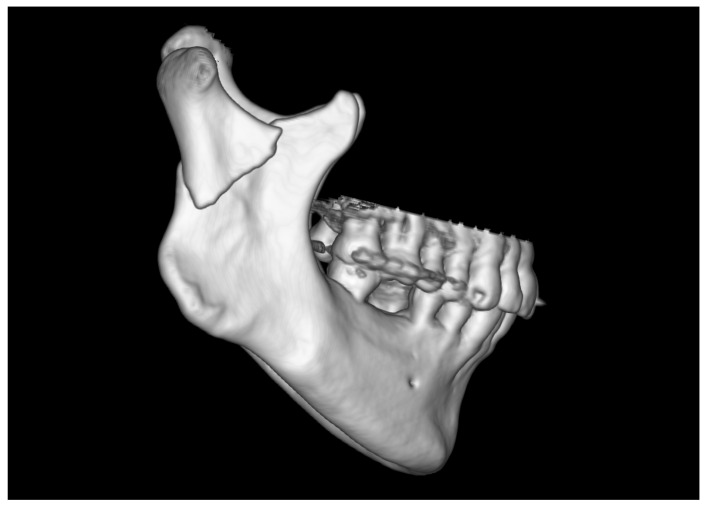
Radiological image from a multi-row CT scan. The examination was performed on the day of admission to the hospital. A fracture of the base of the right condylar process of the mandible with displacement (i.e., overlap of fragments in fracture site) was observed.
